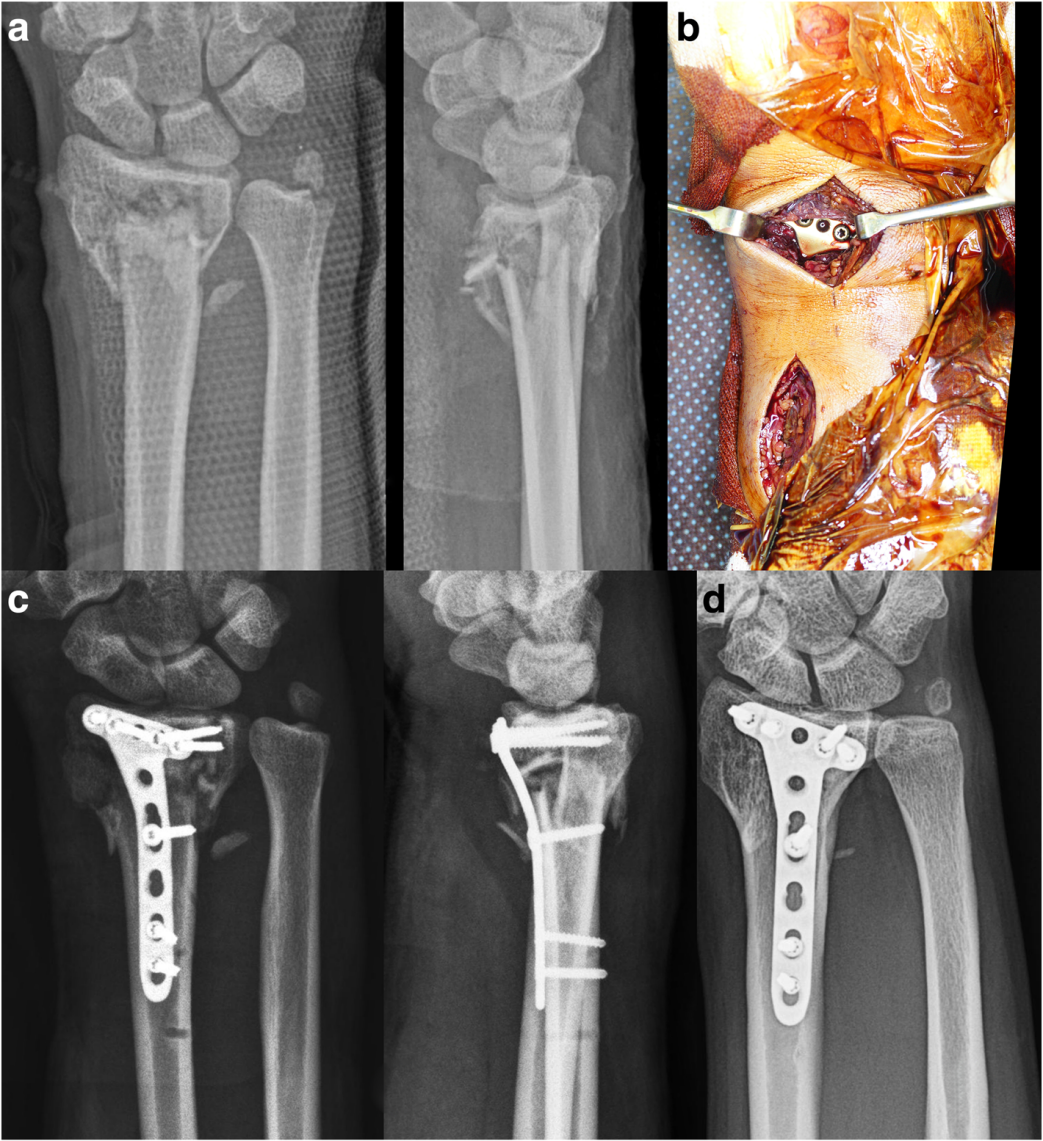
a A 34-year-old man fell from a height of 5 m. Simple radiographs showed metaphyseal comminution in both cortices. b Bridge plating through a PQ-sparing approach was performed with a distractive technique to fix a single volar plate. c Postoperative radiographs showing the restoration of radiologic parameters, including radial length, volar tilt, and radial inclination. d Radiographs after 24 months showing sound union without complications
